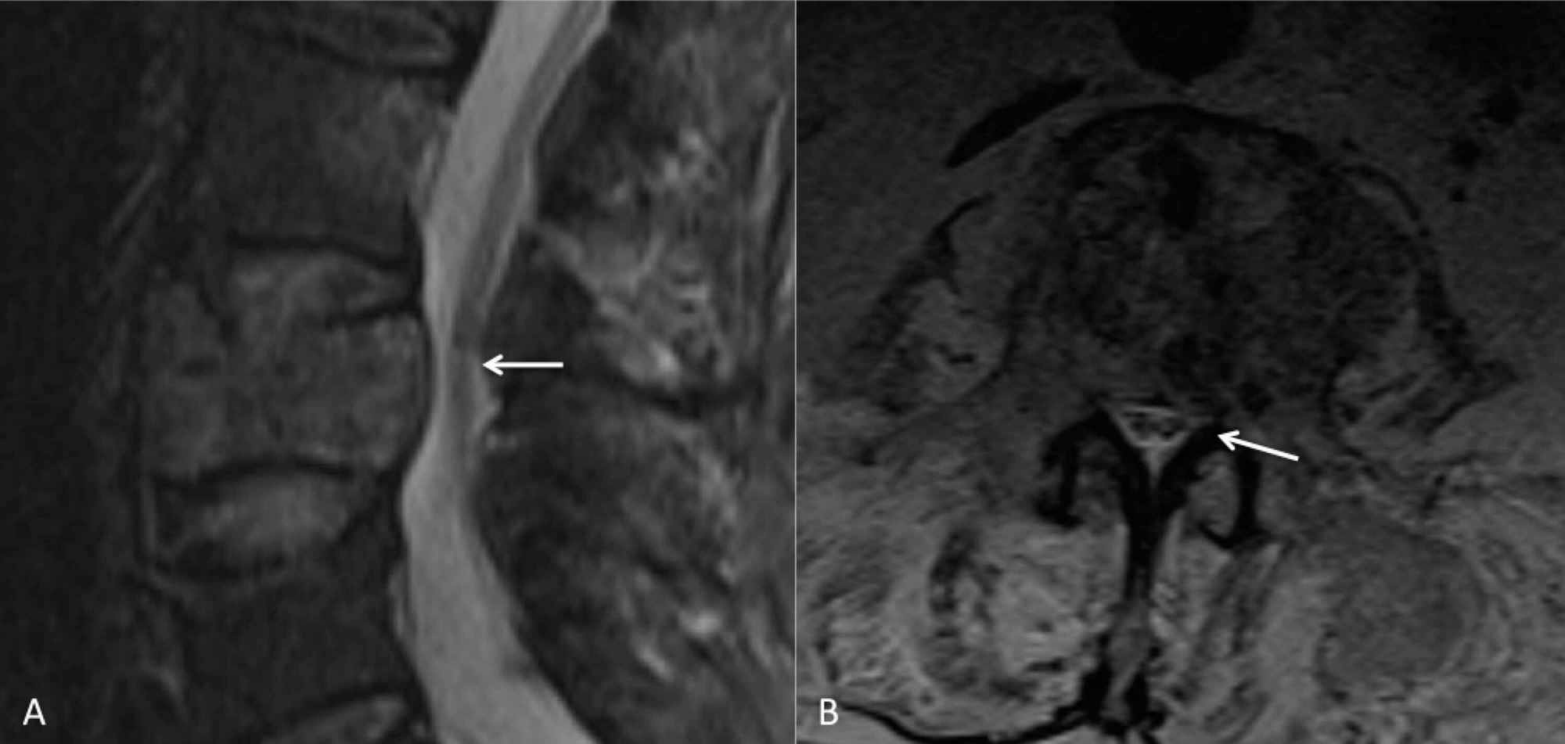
Post-treatment sagittal STIR-weighted (A) and axial T2-weighted (B) images demonstrating reduced tumour volume, resolution of epidural disease and improved canal dimensions (white arrow). STIR, short TI inversion recovery
